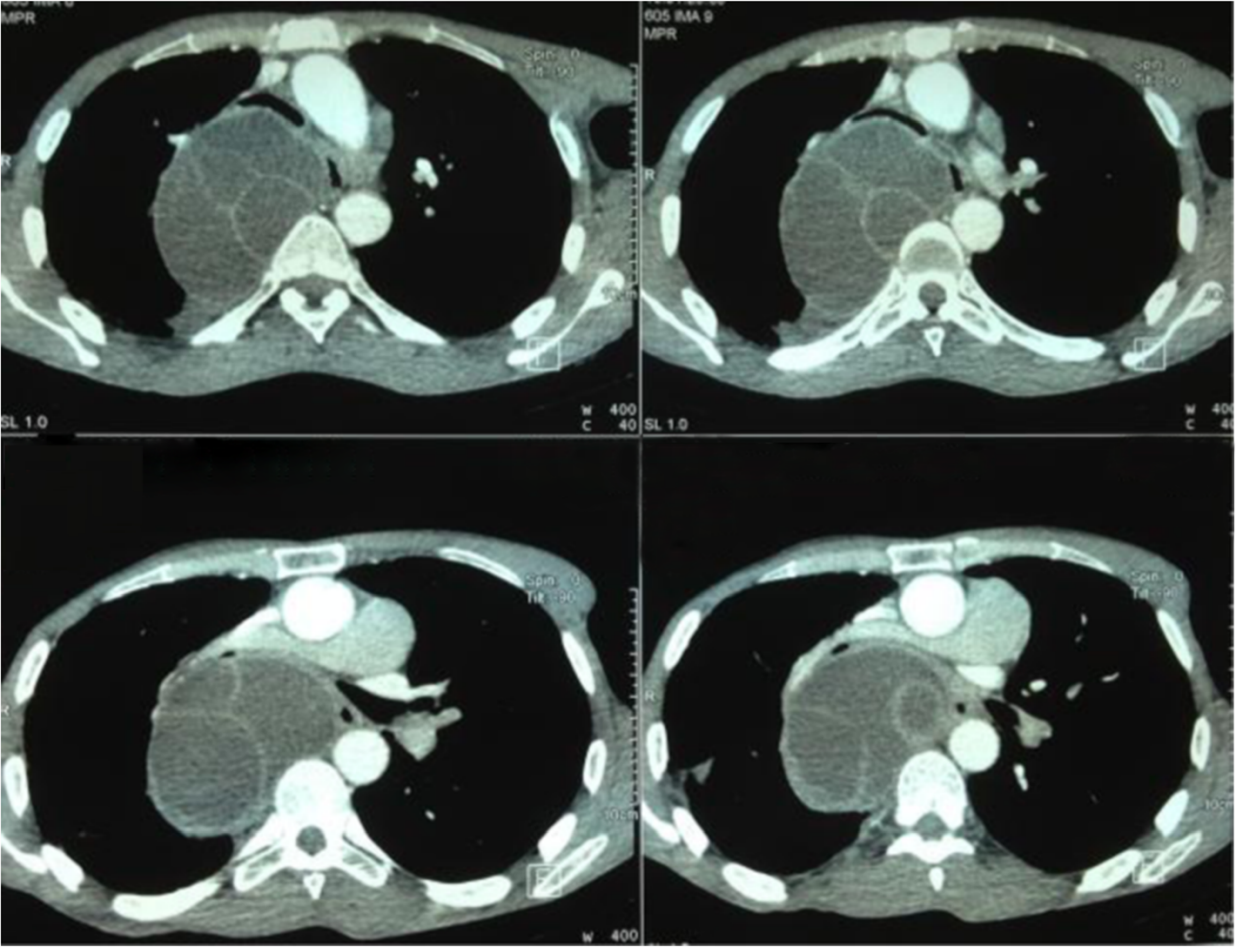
Chest computed tomography after injection of contrast, objectifying a liquid formation in the laterovertebral middle mediastinum with regular contours and fine wall discreetly enhancing by the contrast.
